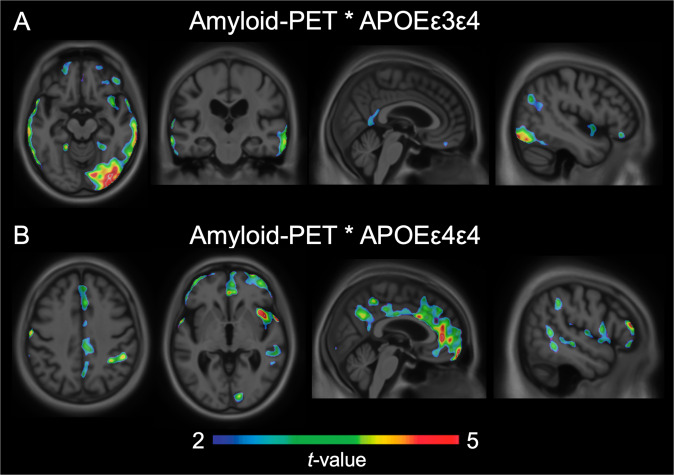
APOEε4 exerts a gene-dose effect on tau aggregation when interacting with amyloid-β. a The interaction between [^18^F]Florbetapir and a single *APOEε4* gene was related to increased [^18^F]Flortaucipir uptake in the posterior cingulate posterior parietal, lateral temporal temporooccipital, and orbitofrontal cortices. b Homozygous *ε4* carriers demonstrated a more widespread relationship between [^18^F]Florbetapir and [^18^F]Flortaucipir uptake, with [^18^F]Flortaucipir uptake in the posterior cingulate, precuneus, posterior parietal, medial prefrontal, and orbitofrontal cortices. Tau-PET uptake in the temporooccipital cortex was observed only for the interaction between [^18^F]Florbetapir SUVR and one *APOEε4* allele. Effects of homozygosity were observed in the tau-PET uptake in the precuneus, anterior cingulate, and medial prefrontal cortices were observed only for the interaction between [^18^F]Florbetapir SUVR and two *APOEε4* alleles. T-statistical parametric maps were corrected for multiple comparisons using a Random Field Theory cluster threshold of *P* < 0.005, overlaid on the ADNI reference template. Age, clinical diagnosis, and amyloid-β SUVR were employed as covariates in each model. Results remained comparable when using partial volume corrected PET data.
